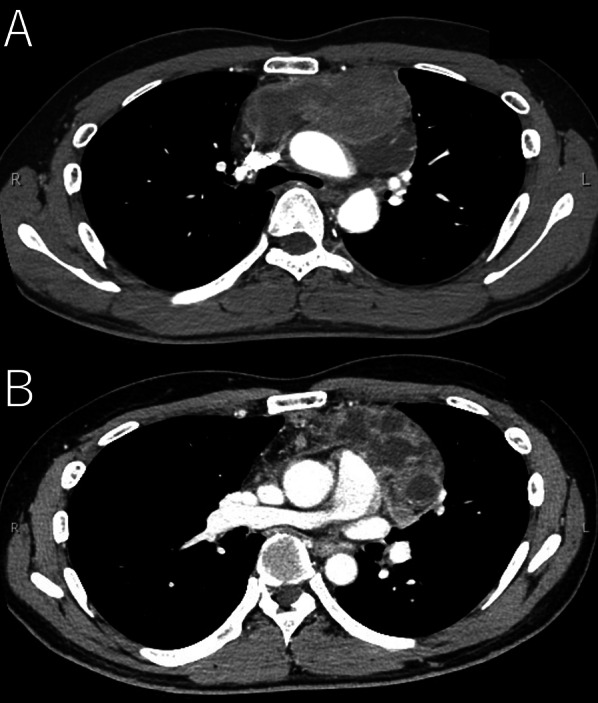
CE-CT images showing huge tumor in anterior mediastinum. A Compressed trachea and esophagus. B Suspected invasion to main trunk of pulmonary artery, without distal metastasis
